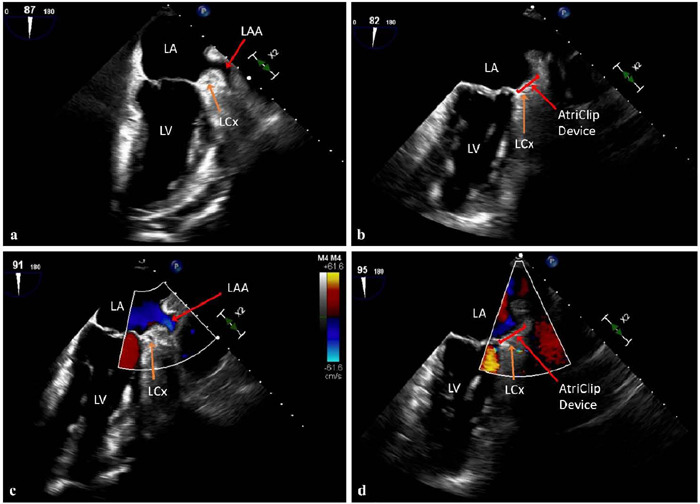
Intraoperative transesophageal echocardiogram with Doppler. Patient D
